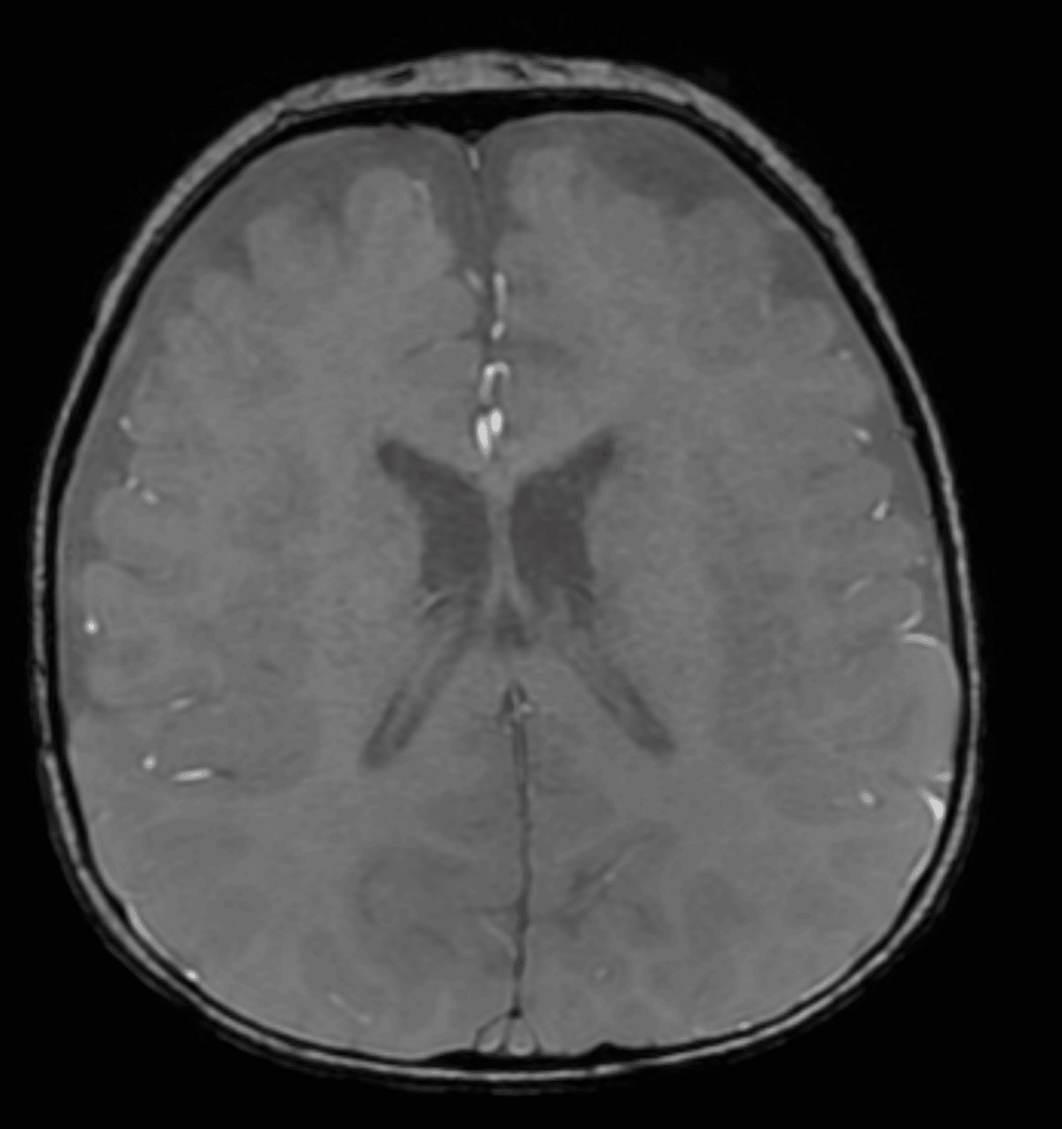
Brain MRI (Axial SWAN) performed at eight months of age showing prominent extra-axial CSF spaces without evidence of intracranial hemorrhage or abnormal susceptibility, supporting the diagnosis of BESS SWAN: susceptibility-weighted angiography; CSF: cerebrospinal fluid; BESS: benign enlargement of subarachnoid spaces
